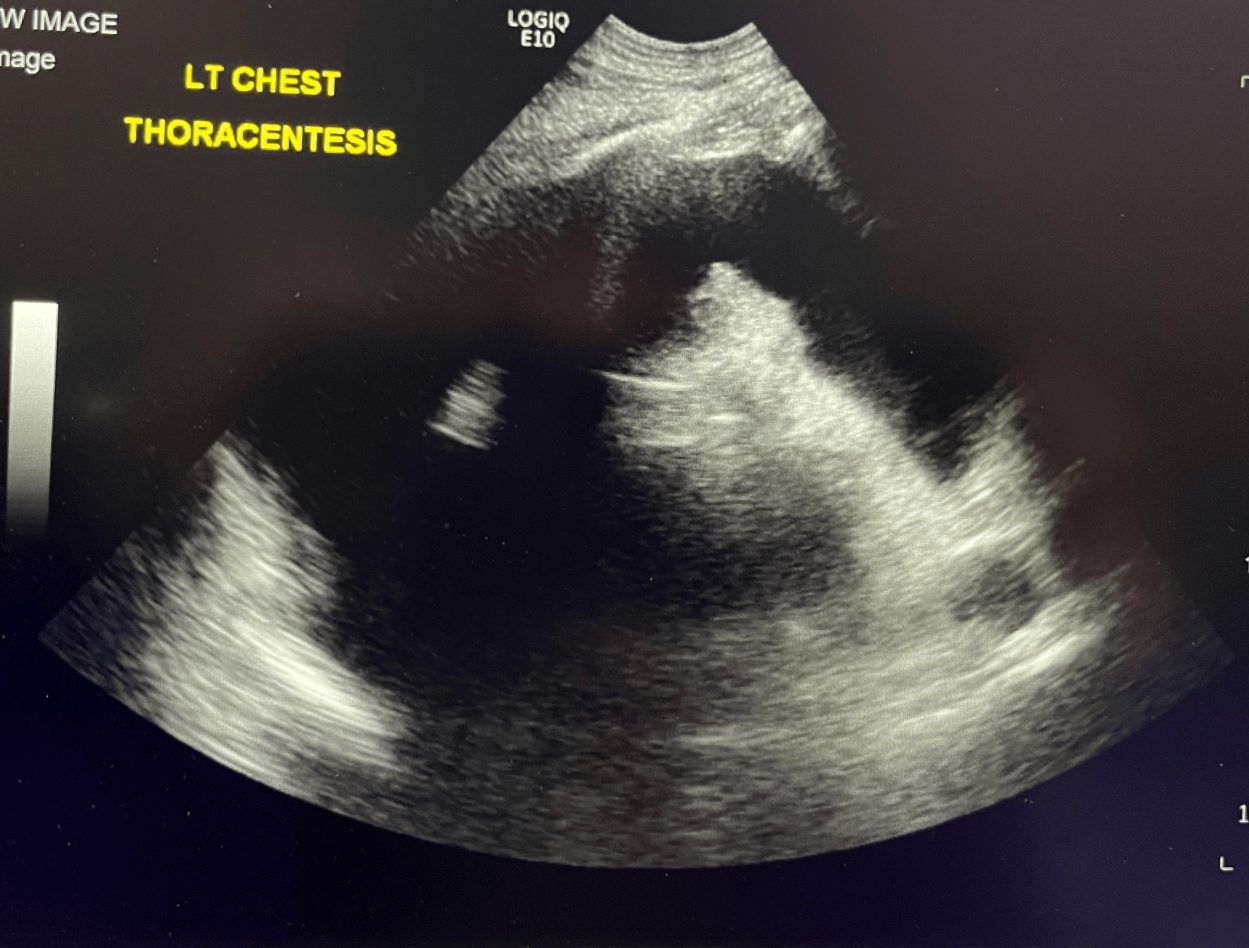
Postoperative ultrasound of the pleural space following interventional radiology drainage
